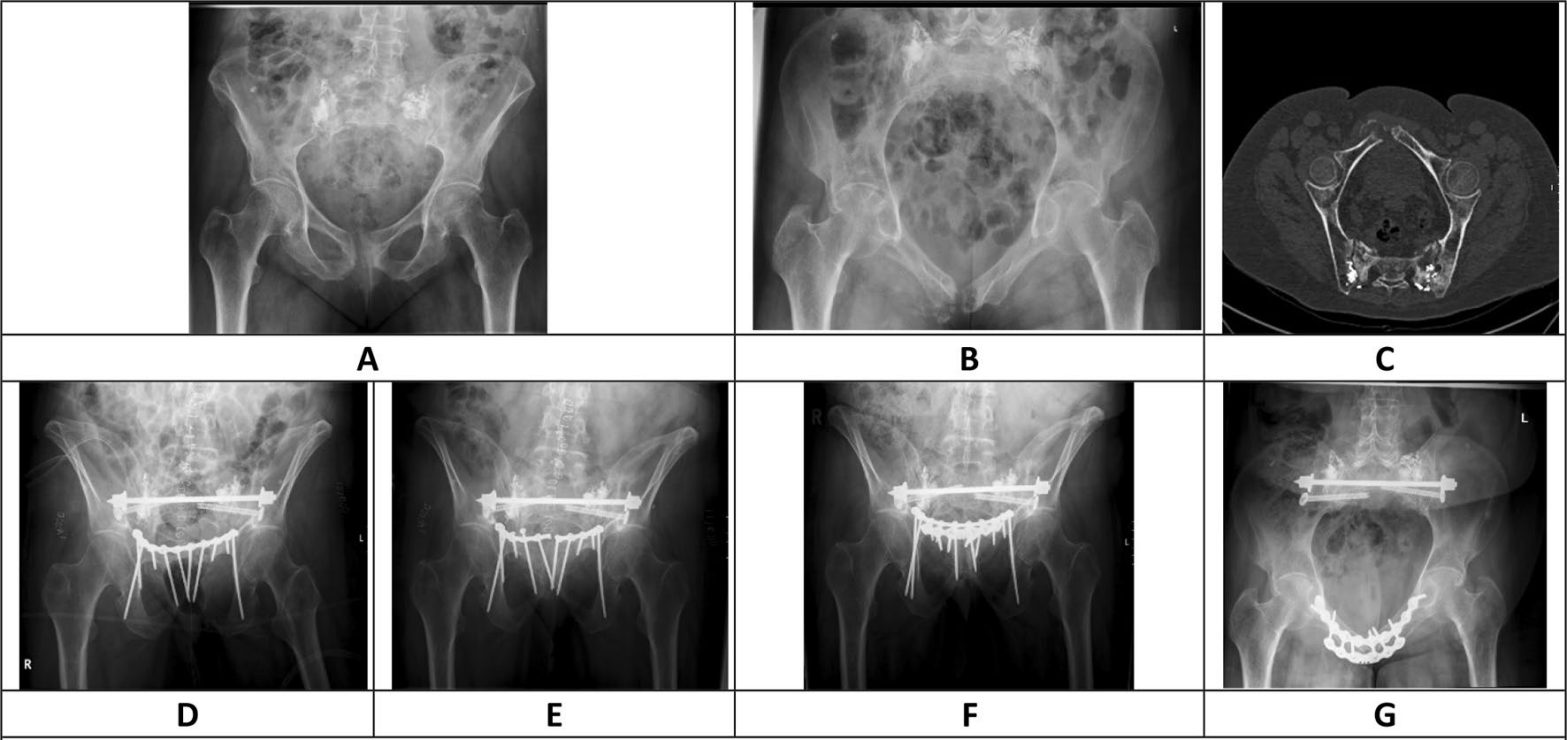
Shows a 67 year old patient who was treated with a sacroplasty elsewhere but transferred to our center due to fracture progression. She presented with a FFP 4c fracture and anterior instability of the pubic symphysis extending into the Nakatani I region 91 days after the injury. (a Conventional ap radiograph, b conventional inlet radiograph, c CT reconstruction in pelvic brim view). The fracture of the anterior pelvic ring was located at the level of the symphysis and extending into the right Nakatani I region. The chronic fracture was initially treated with a sacral bar and bilateral SI screws posteriorly and a ten-hole SPO with bilateral infra-acetabular screws. (d Immediate post-operative conventional outlet radiograph). The plate broke 9 days after the initial operation (e 10 day post-operative conventional outlet radiograph) and a revision DPO was performed with an additional anterior six-hole angular stable plate. A follow-up of 4 months was available and during this time period no screw loosening was observed. (f Conventional outlet radiograph 4 months post-operative, g conventional inlet radiograph 4 months postoperative)
